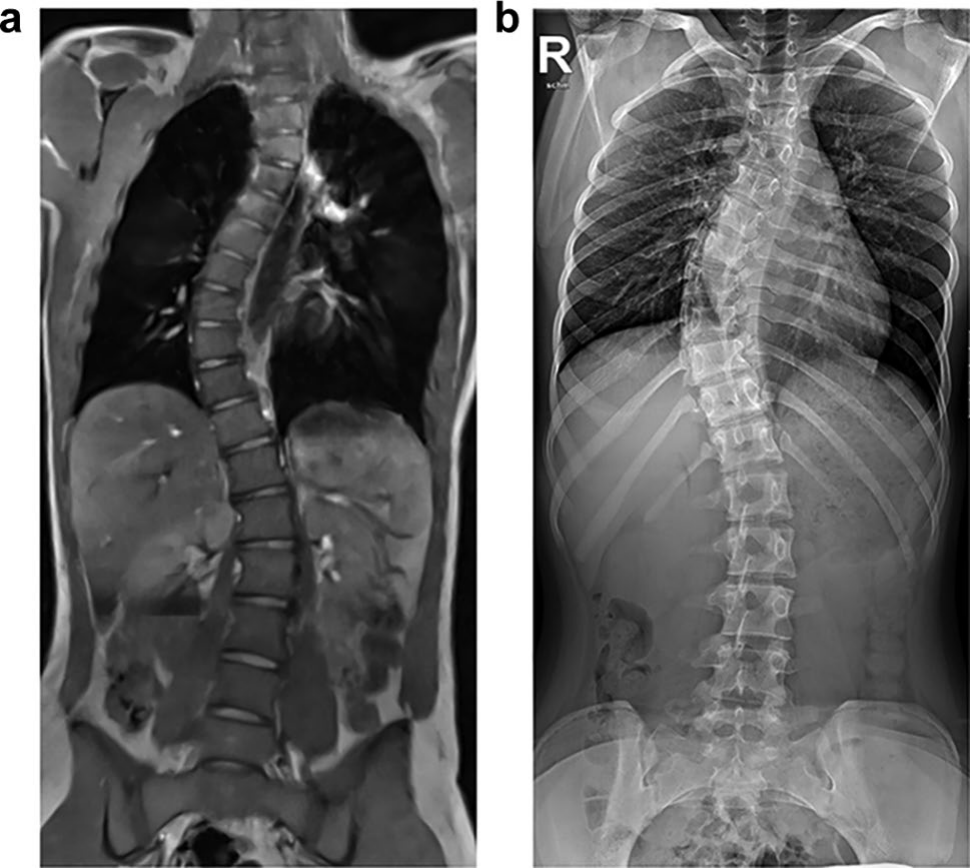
15-year-old girl with juvenile scoliosis. a Curved multiplanar reconstruction of a composed volume coverage real-time MRI sequence. b Corresponding conventional, stitched X-ray of the spine
